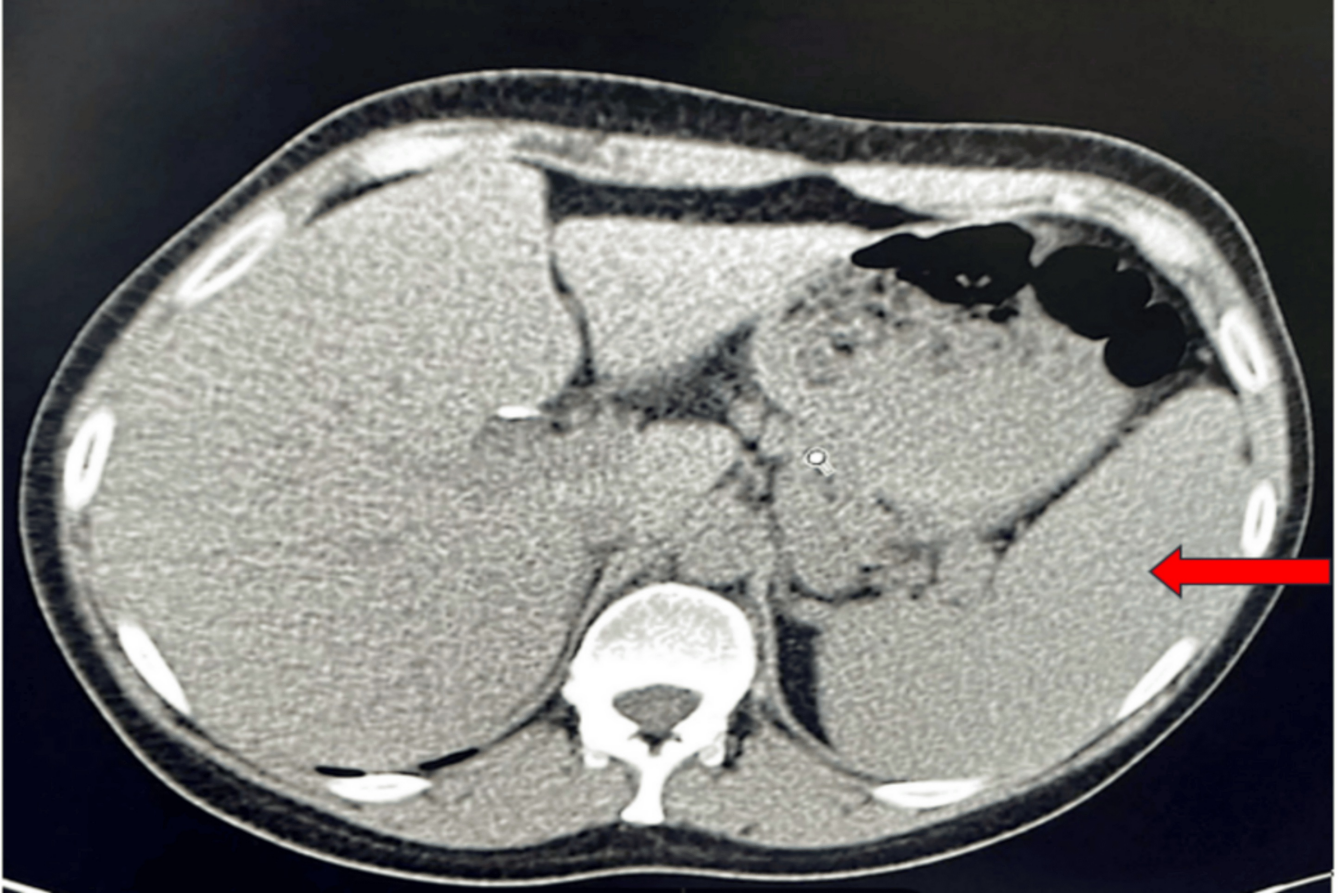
Abdominal CT scan showing splenomegaly. Red arrow: Splenomegaly measuring 115 mm in size with homogeneous density.
